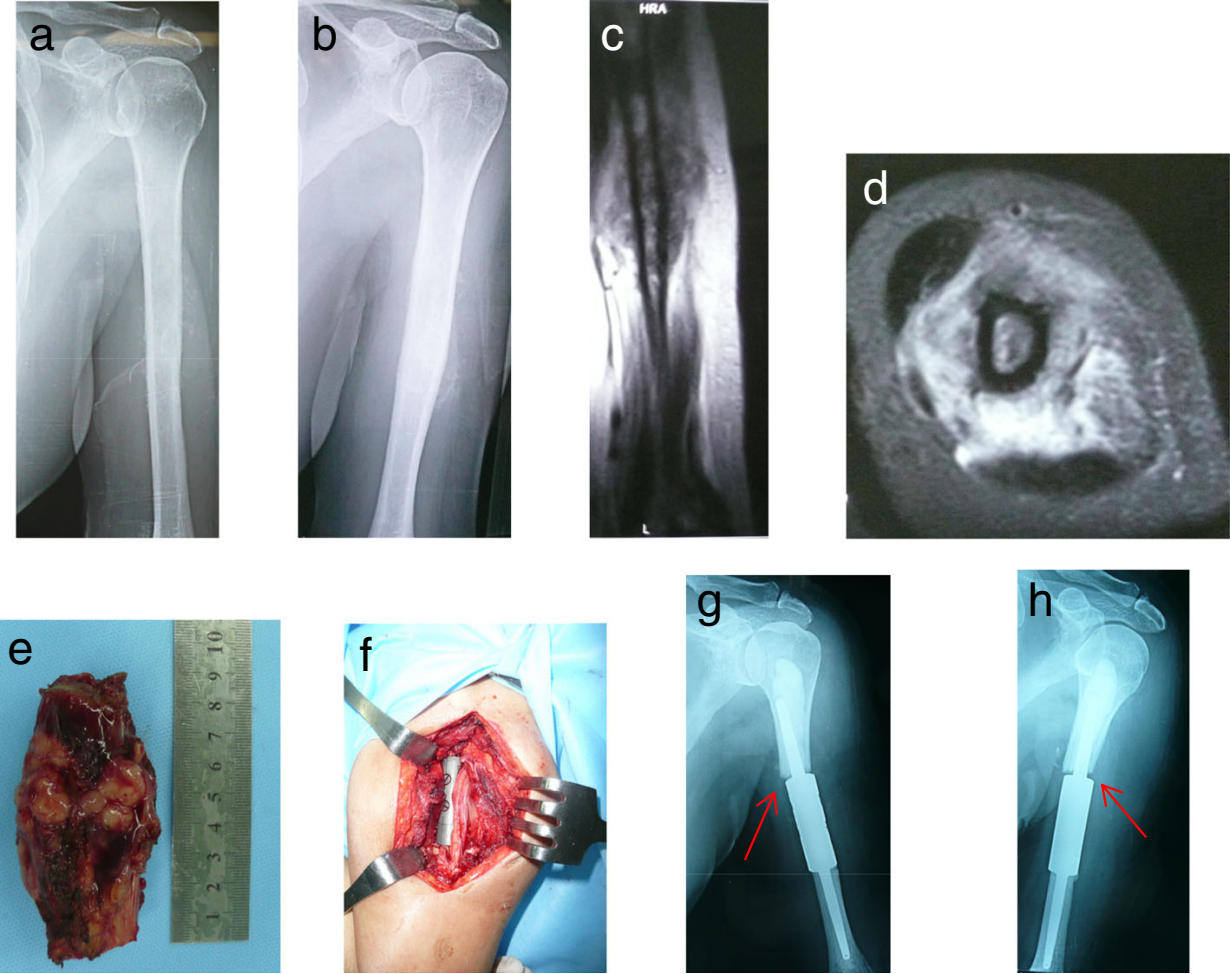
Case 23, a 65-y old female patient with metastatic tumor secondary to breast cancer. The bone strength score is 12. a, b The preoperative X-ray showed that the osteolytic destruction was located in the diaphysis of left humerus(S5 region). c, d The preoperative MRI indicated that the intramedullary destructive signals and soft-tissue swelling. e, f This patient underwent resection of the tumor and intercalary prosthetic reconstruction. g, h The proximal stem of the prosthesis occurred aseptic loosening 3 months after operation(arrow)
